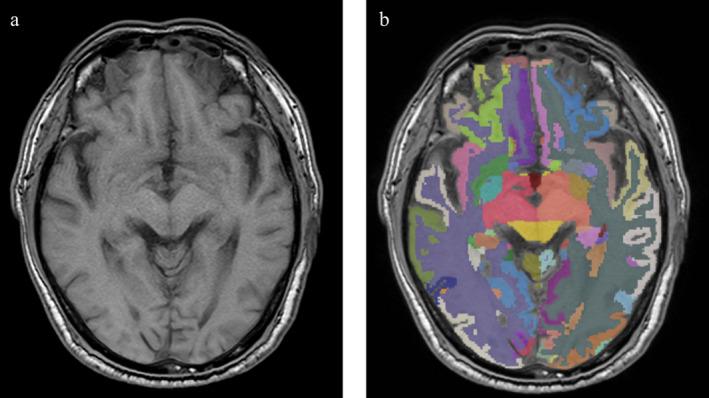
Quantitative steps of the main major cerebral cortex and subcortical nuclei. (a) Original MRI image. (b) The analysis interface of the uAI Research Portal.
